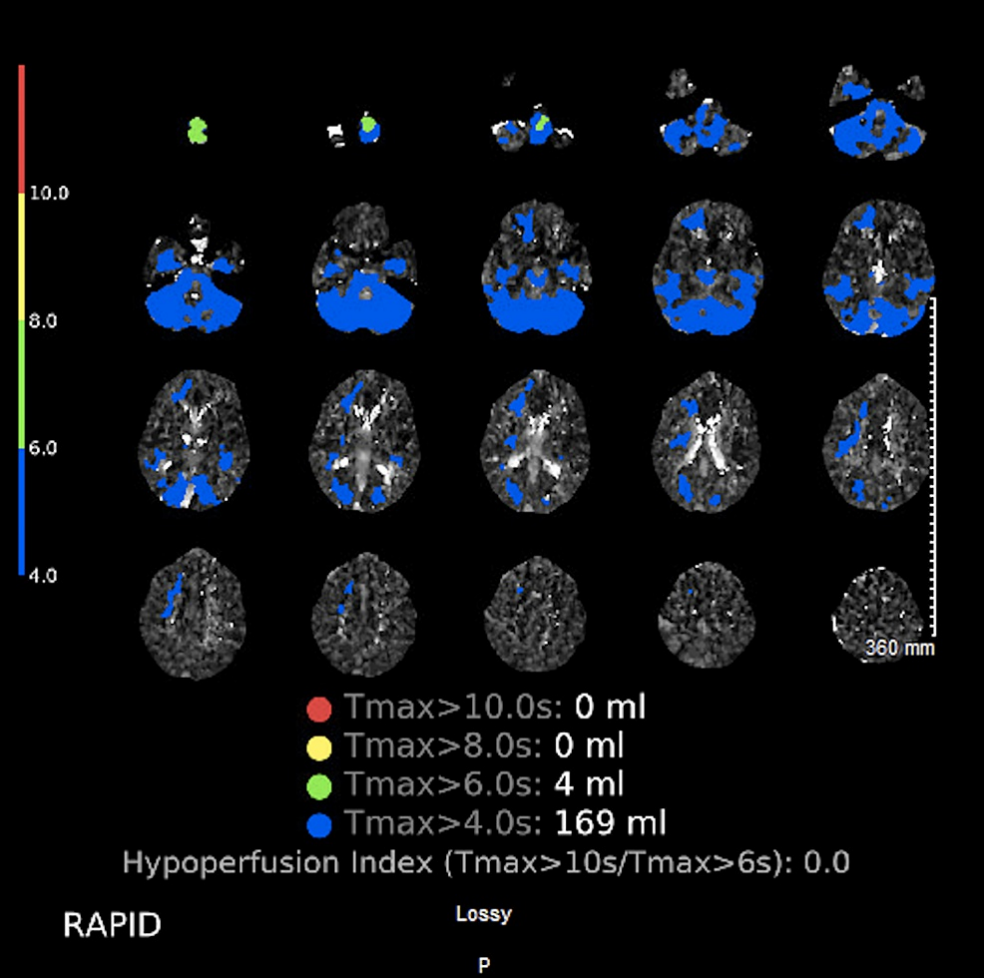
CT perfusion brain T-max values
